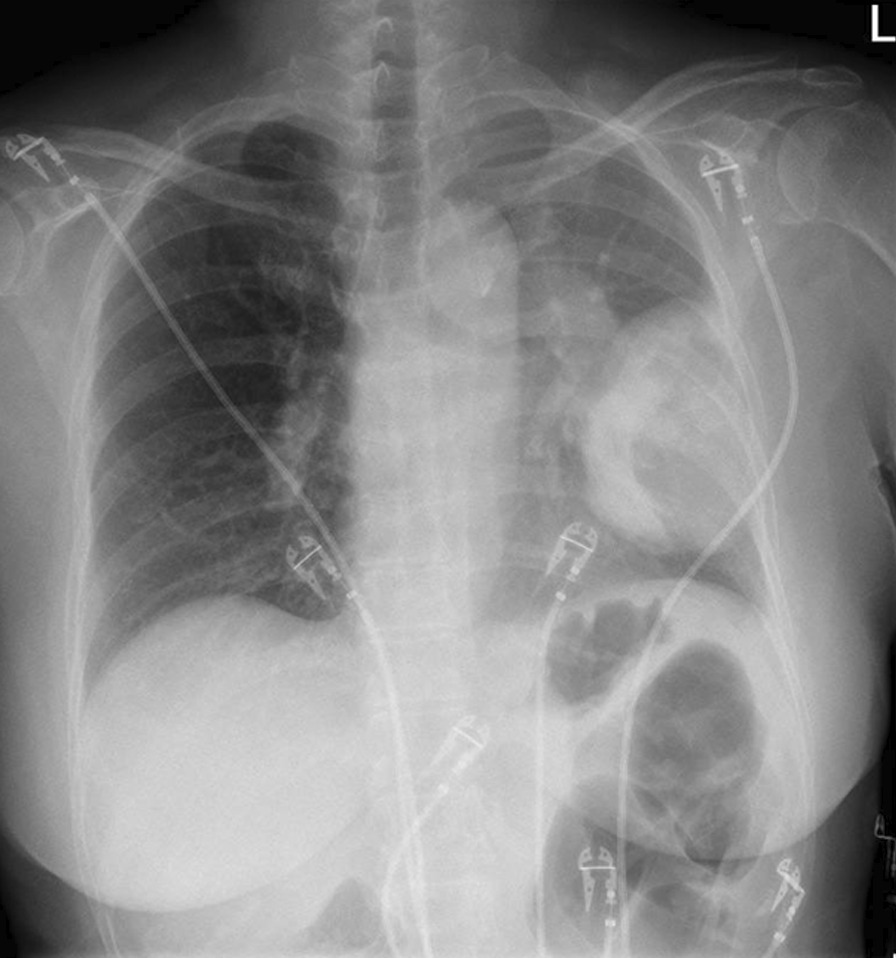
Chest radiograph of patient on diagnosis of non-small cell lung carcinoma
